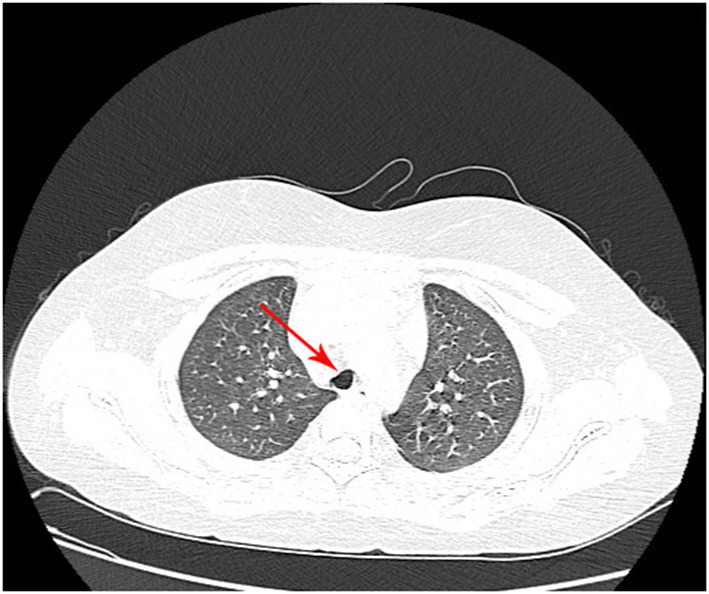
Chest computed tomography at 1 year postoperatively shows that the anterior wall of the trachea has collapsed slightly.
